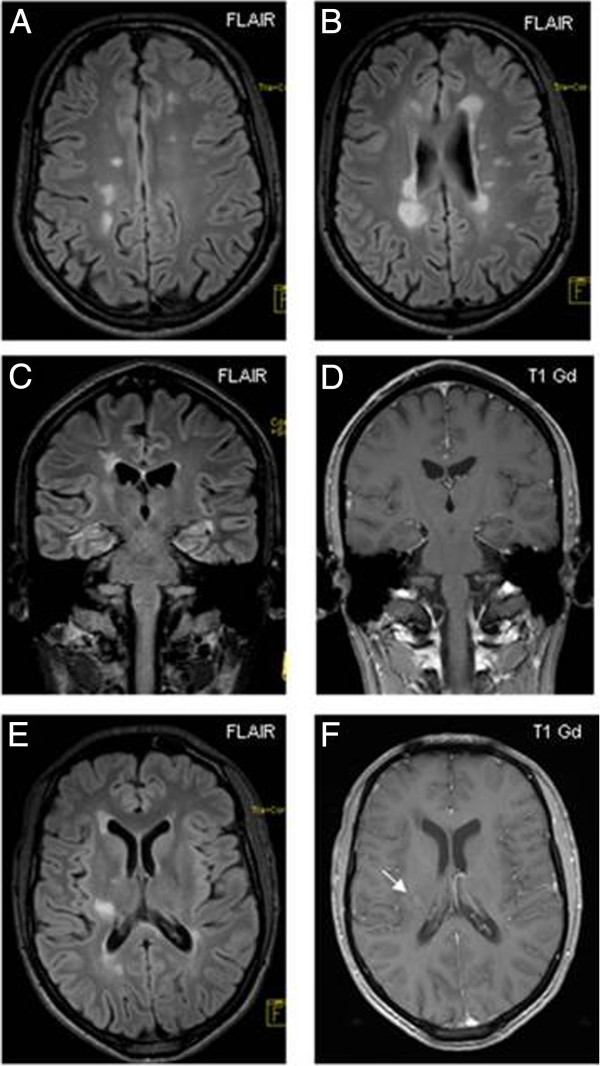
Magnetic resonance imaging. MR FLAIR imaging demonstrates multiple hyperintense lesions suggestive of multiple sclerosis (A-C, E). One of the lesions (arrow) was found to be Gadolinium (Gd) enhancing on T1 scans (D, F).
